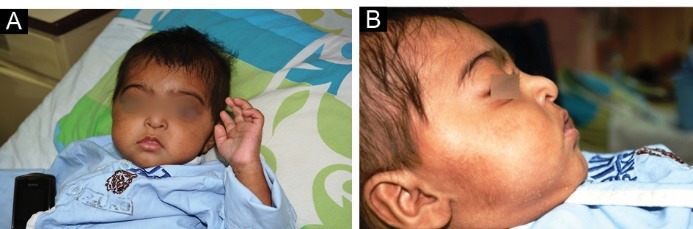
Ultrasound abdomen showed moderate ascites and no hepatosplenomegaly. Both kidneys were normal in size.
